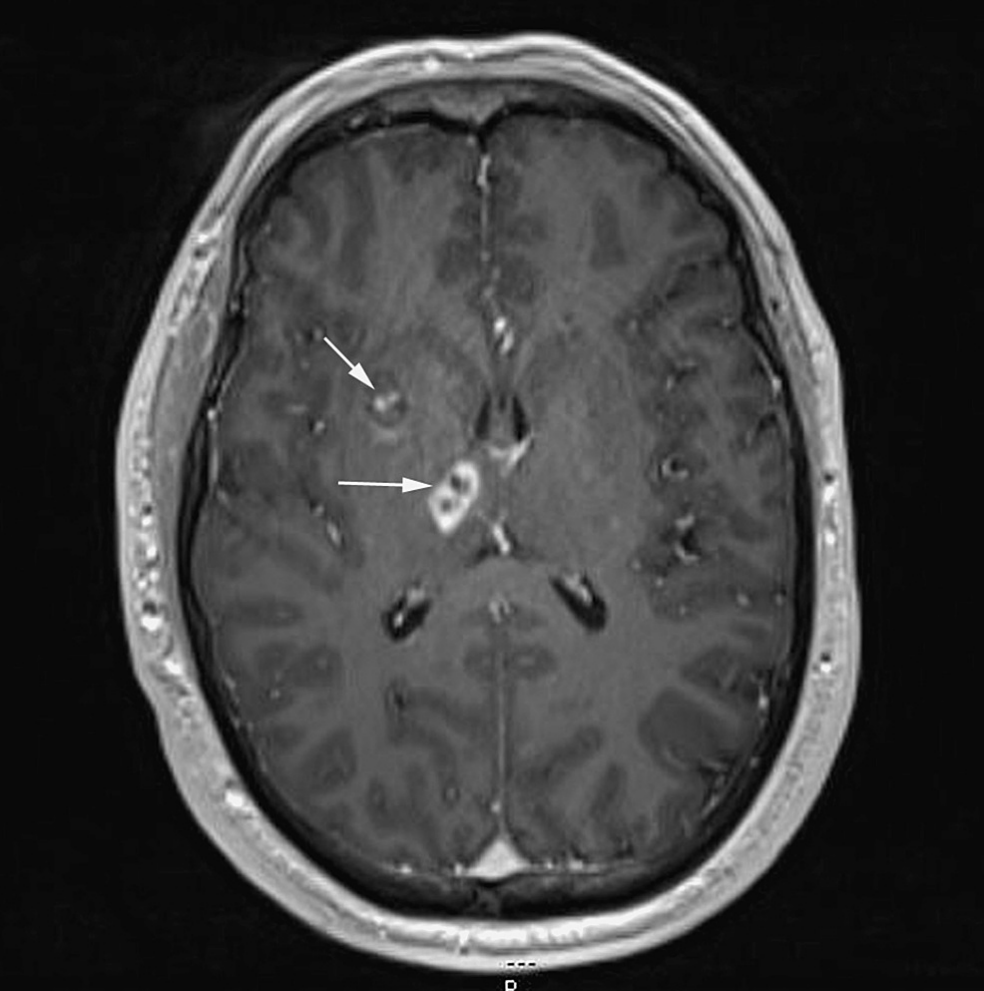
MRI Brain Arrows show lesions of the right basal ganglia and right thalamus which could represent subacute lacunar infarcts MRI (Magnetic resonance imaging)
